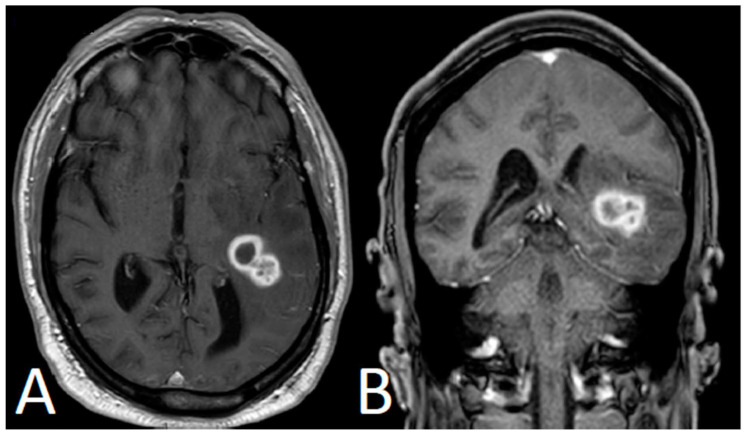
Brain MRI showing lobulated left temporal mass with peripheral enhancement with surrounding cerebral edema (A: axial view; B: coronal view).
